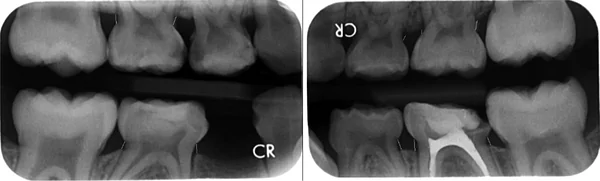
Radiographs of an 8‐year‐old patient describing digital measurements by tracing a cementoenamel junction and bone crest (CEJ‐BC) line using the Image J software.
